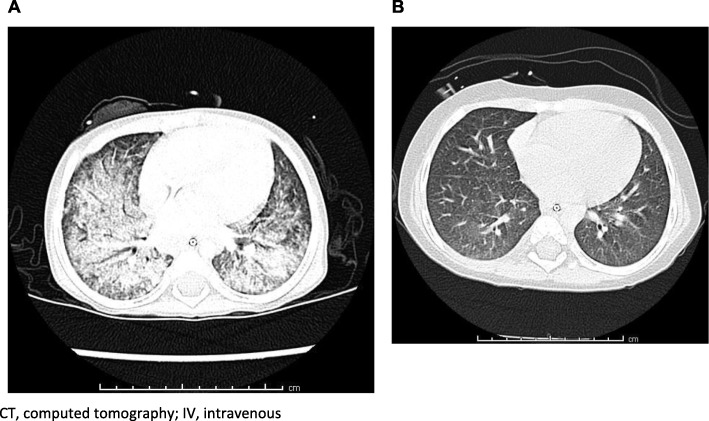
CT scans for Patient SEQ7 prior to (a) and after 9 months of treatment (b) with IV HPβCD. CT, computed tomography; IV, intravenous
